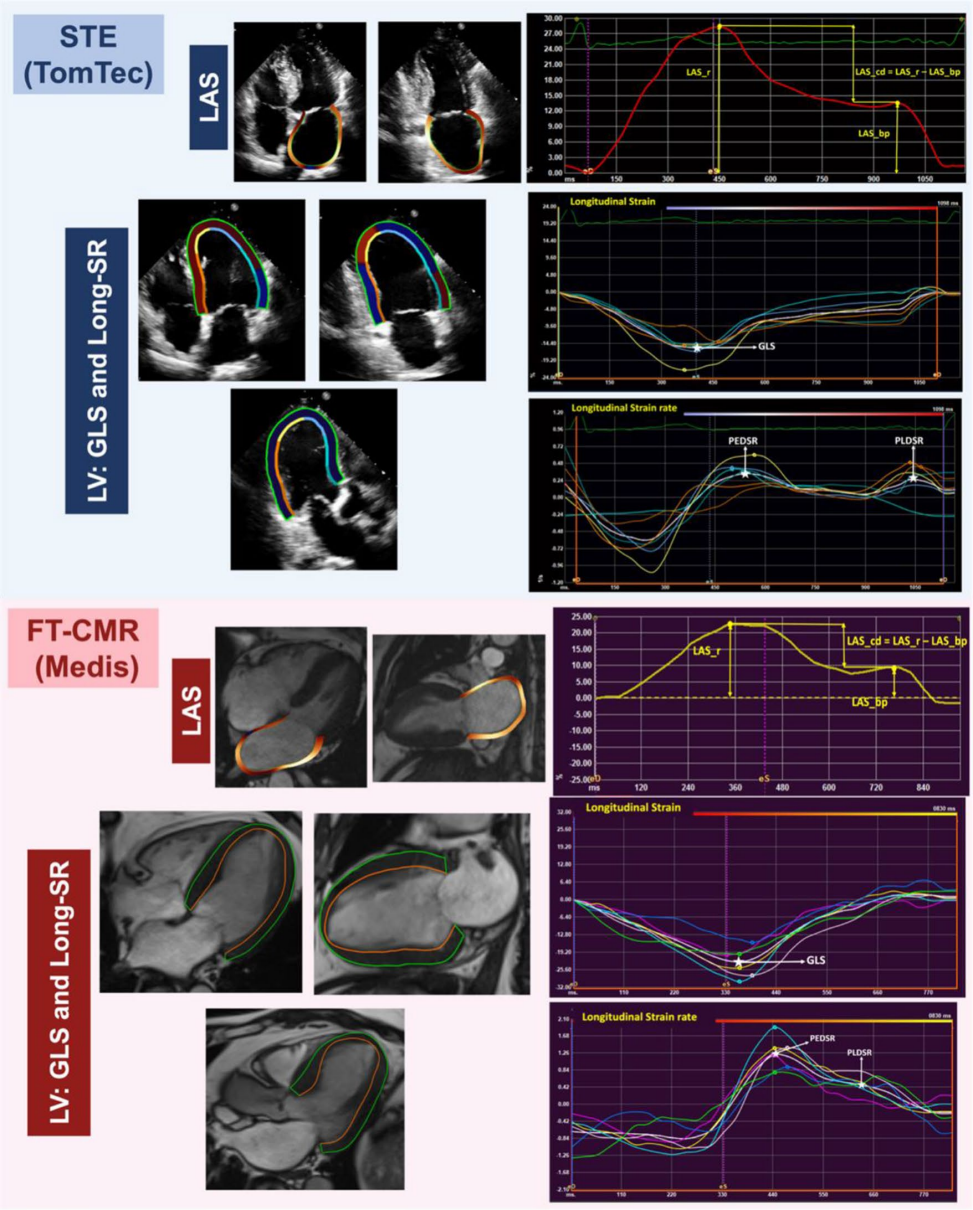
LA and LV longitudinal strain and LV diastolic strain rate analysis using Speckle Tracking Echocardiography (Upper) and Feature tracking-CMR (Lower). Abbreviations: STE = Speckle Tracking Echocardiography, LAS_r/cd/bp = Left atrial strain during reservoir/conduit/ booster pump phase, GLS = global longitudinal strain, SR = strain rate PE/PLDSR = peak early/peak late diastolic strain rate, FT-CMR = feature tracking– cardiovascular magnetic resonance imaging
